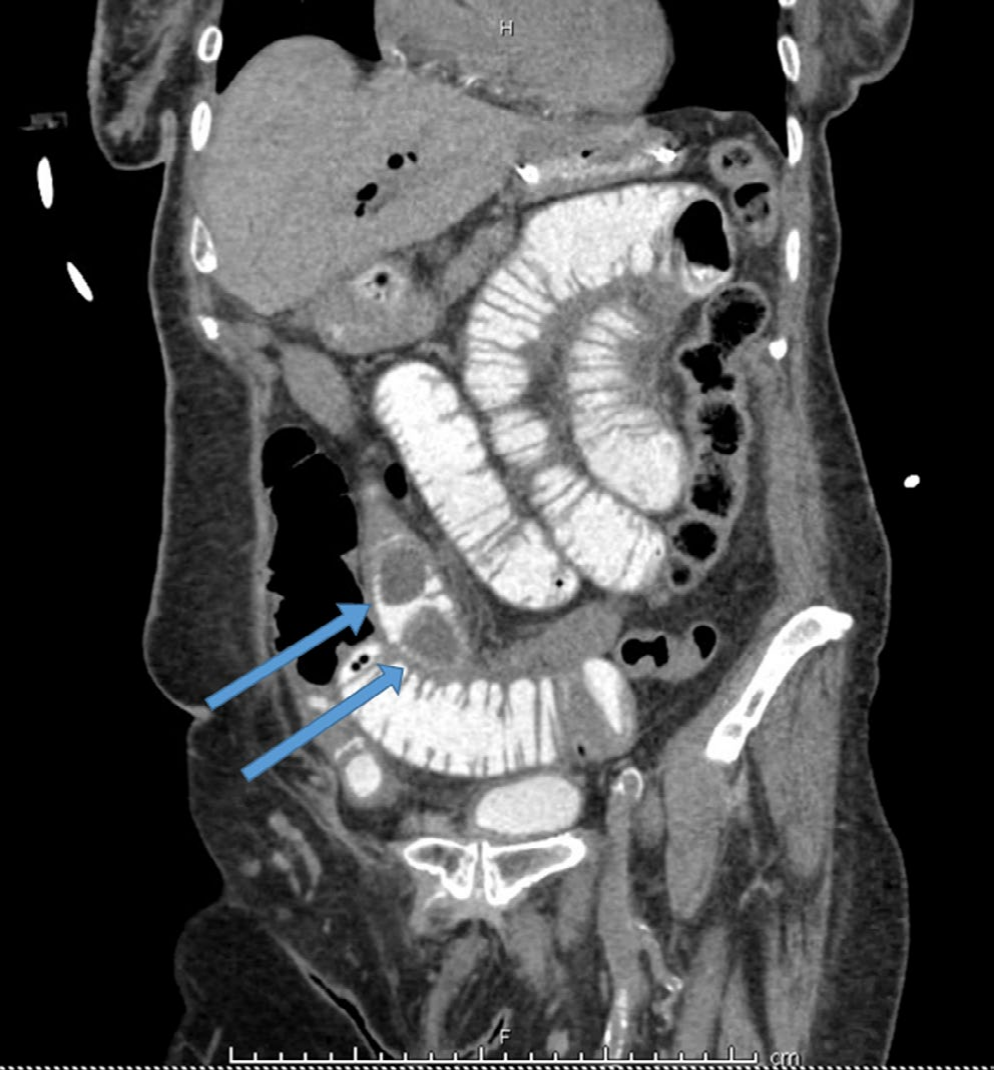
Coronal view of a computed tomography scan of the abdomen and pelvis (enhanced with oral contrast). Arrows point to filling defects resulting from gallstones in the small‐bowel lumen
